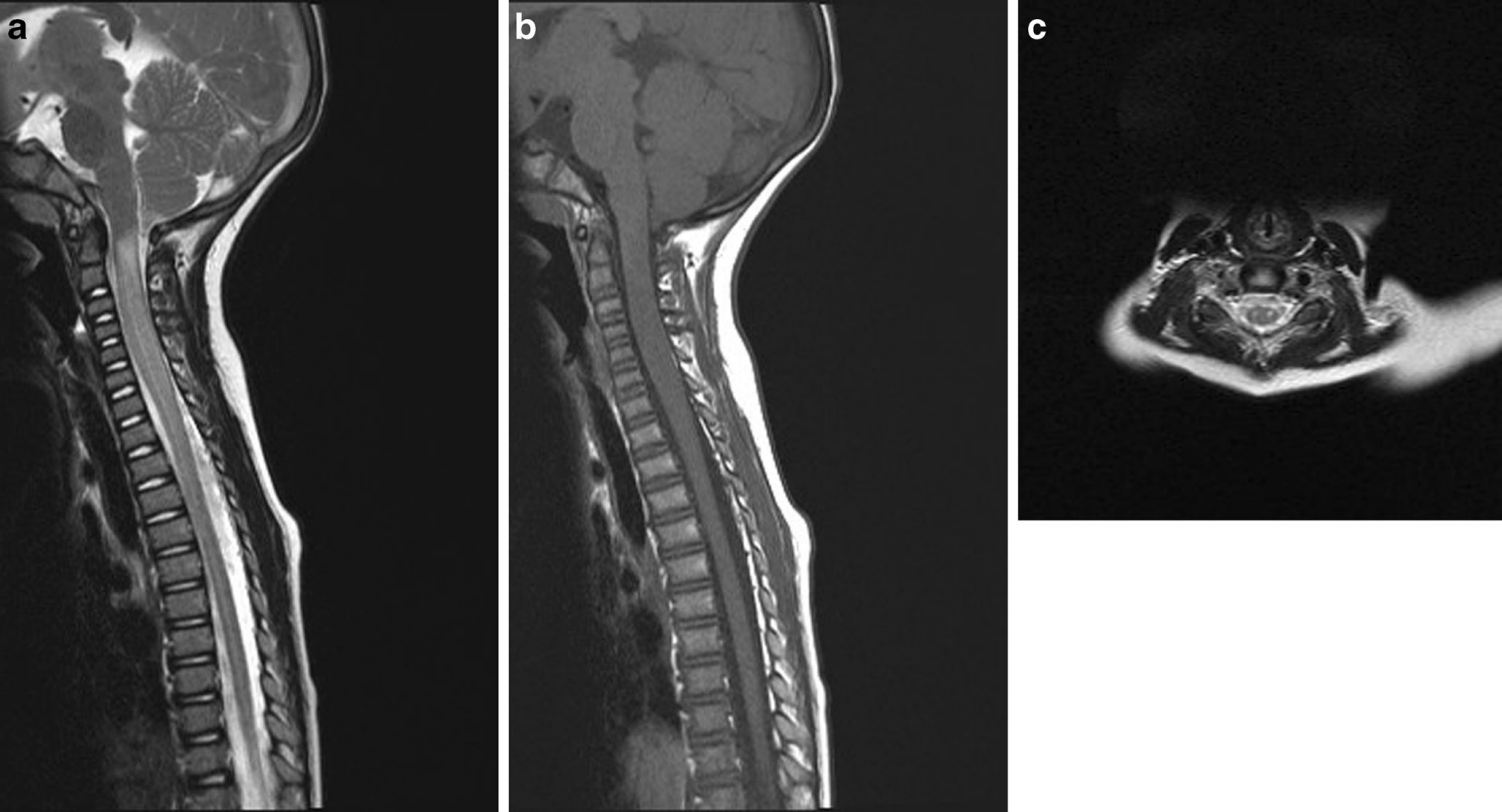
a T2 and b T1 Sagittal MRI of the cervical spine demonstrating diffuse swelling (a, b) and increased T2 signal (a) of the cervical cord substance. Confirmed on axial T2 assessment at the C5 level (c)
